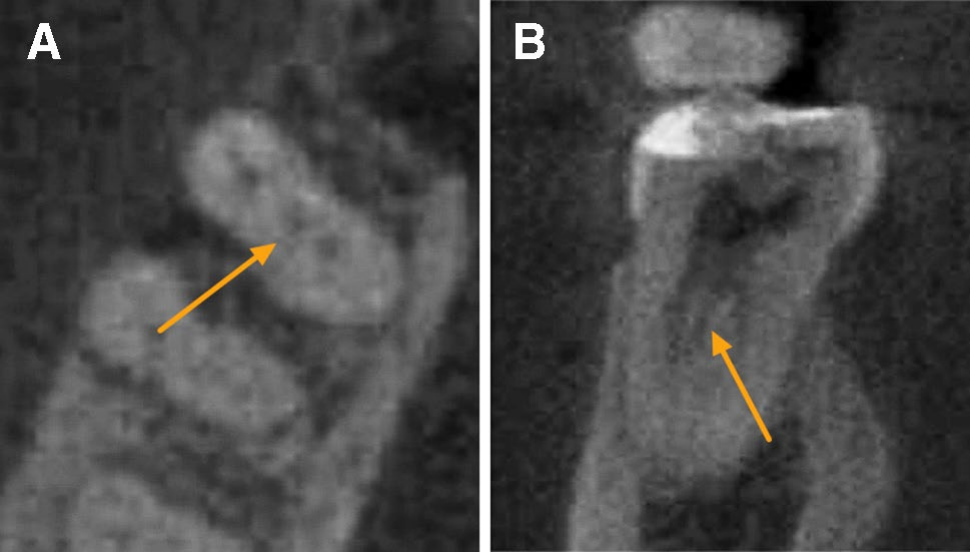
CBCT images showing the middle mesial canal of the mandibular first molar (tooth #30) in the axial (a) and coronal (b) plane. The arrows point to the middle mesial canal
